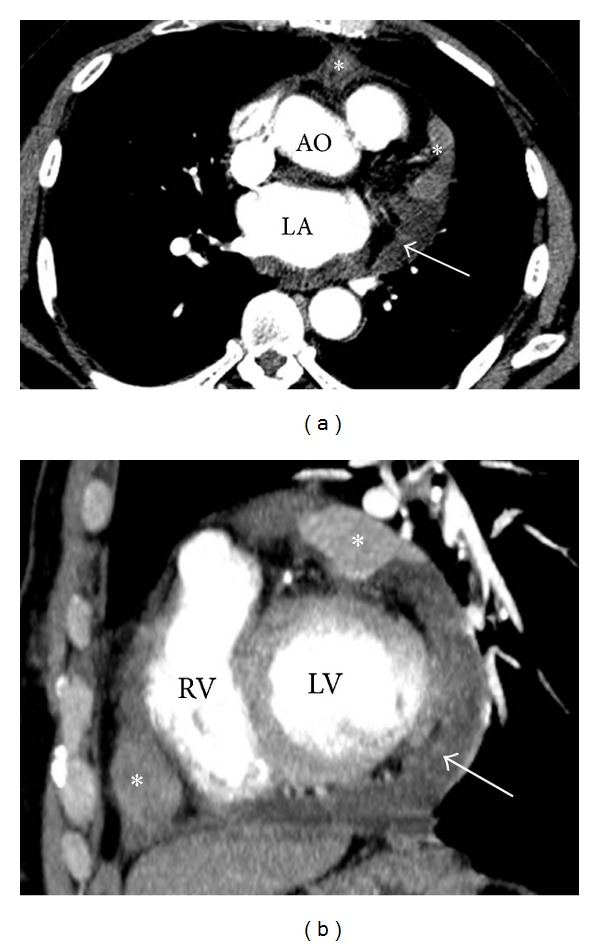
Axial (a) and sagittal (b) contrast-enhanced CT scan showed enhanced soft tissue nodules (asterisk) that have infiltrated the pericardium and moderate amount of pericardial effusion (arrow) (AO: aorta, LA: left atrium, RV: right ventricle, LV: left ventricle).
